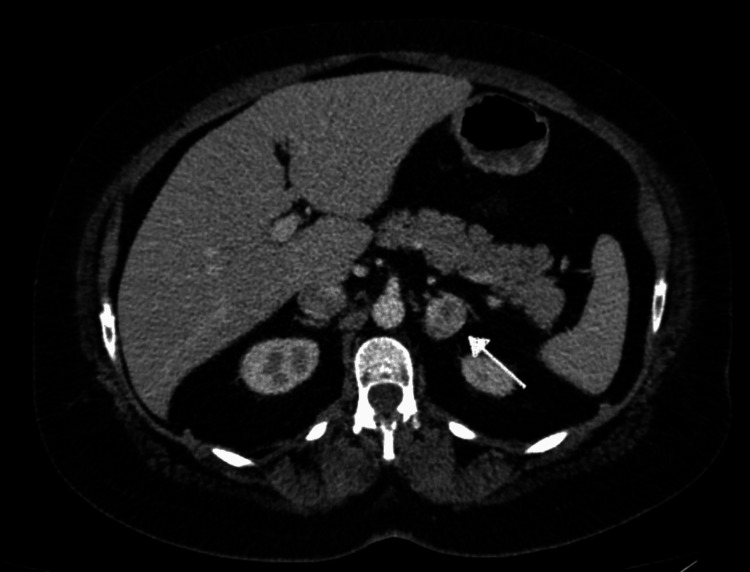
CT abdomen and pelvis revealing a 2.6 cm left adrenal nodule (highlighted by white arrow)
